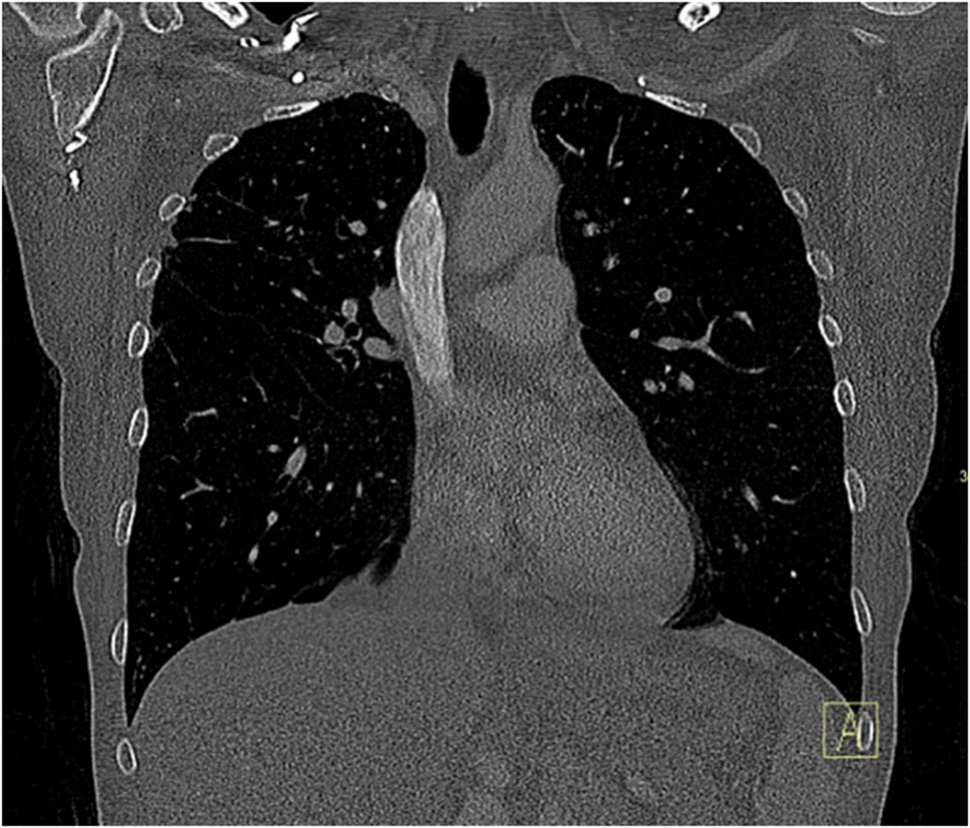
Coronal contrast-enhanced chest CT image showing a widely patent superior vena cava
